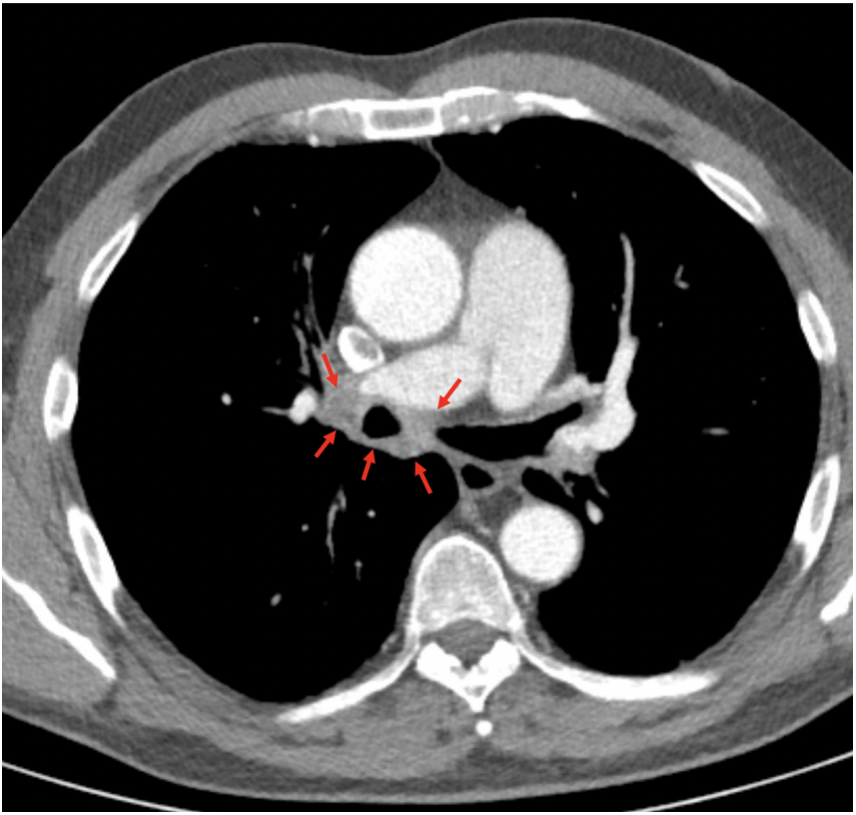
Preinterventional computed tomography showing perihilar soft tissue proliferation circumferentially located around the right main stem bronchus (red arrows).
